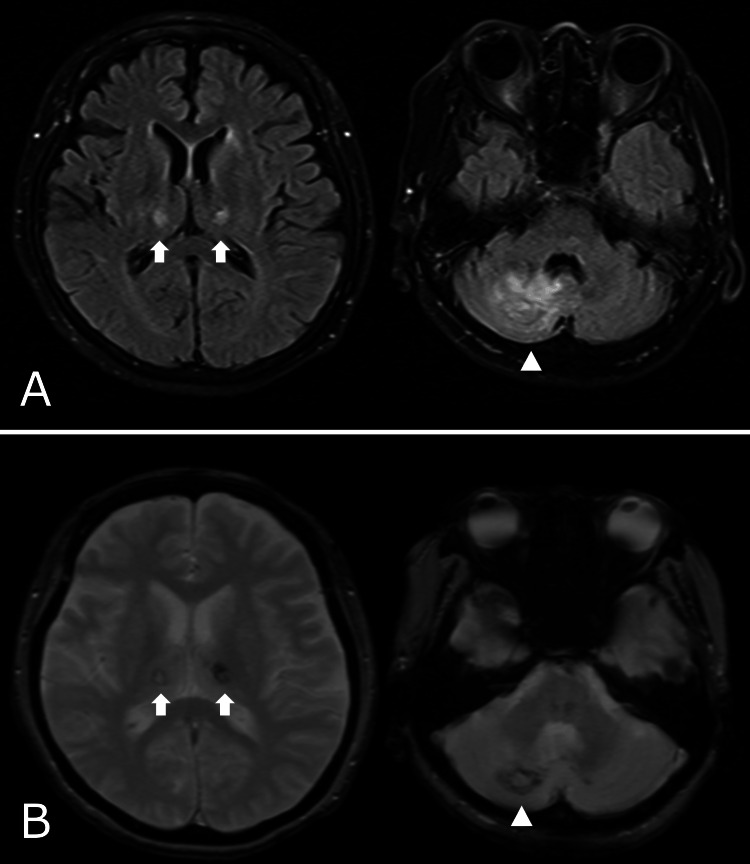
Brain MRI on the day 28. (A) Fluid attenuated inversion recovery imaging showed highly dense areas in the right cerebellar hemisphere (white arrowhead) and bilateral thalamus (white arrow). (B) T2-weighted short-tau inversion recovery imaging showed heterogeneous low-dense areas in the same regions (white arrow and white arrowhead).
